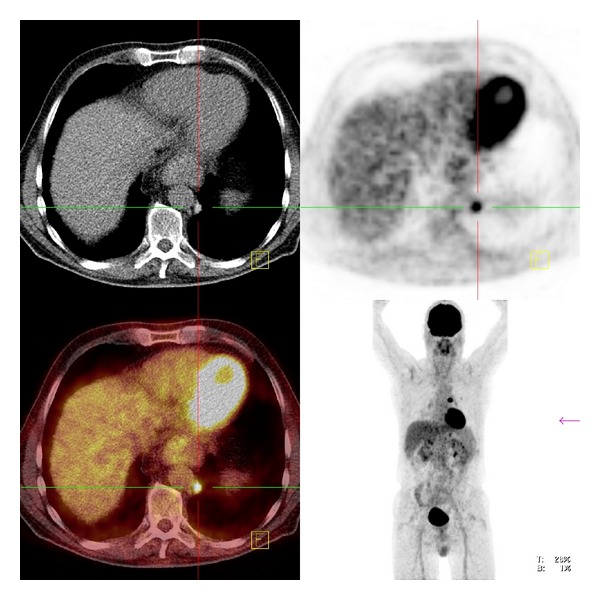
FDG-avid paraaortic nodal structure.
